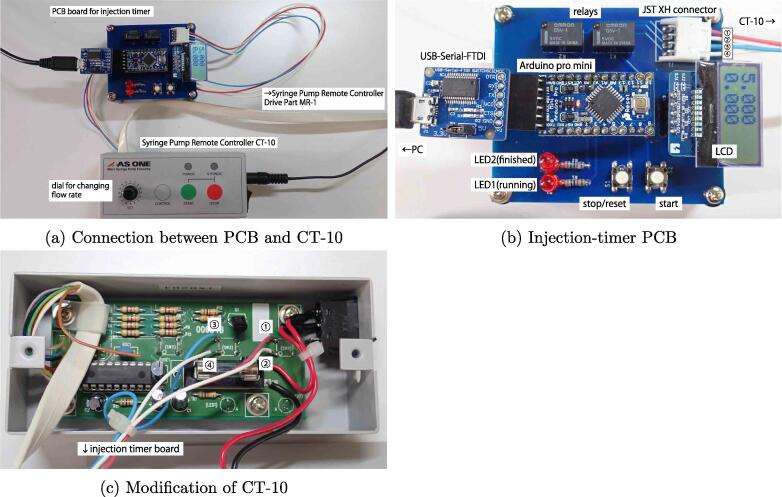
Injection-timer system developed with syringe-pump remote controller CT-10. (a) Arduino controls any relays to switch CT-10 on/ff based on timer state. (b) LCD displays timer set and elapsed times. Two tactile switches can start/stop timer. Two LEDs show running/finished timer states. (c) PCB and CT-10 are connected by four wires. Wires 1 and 2 and wires 3 and 4 are soldered at SW3 and SW4 on CT-10 PCB, respectively.
